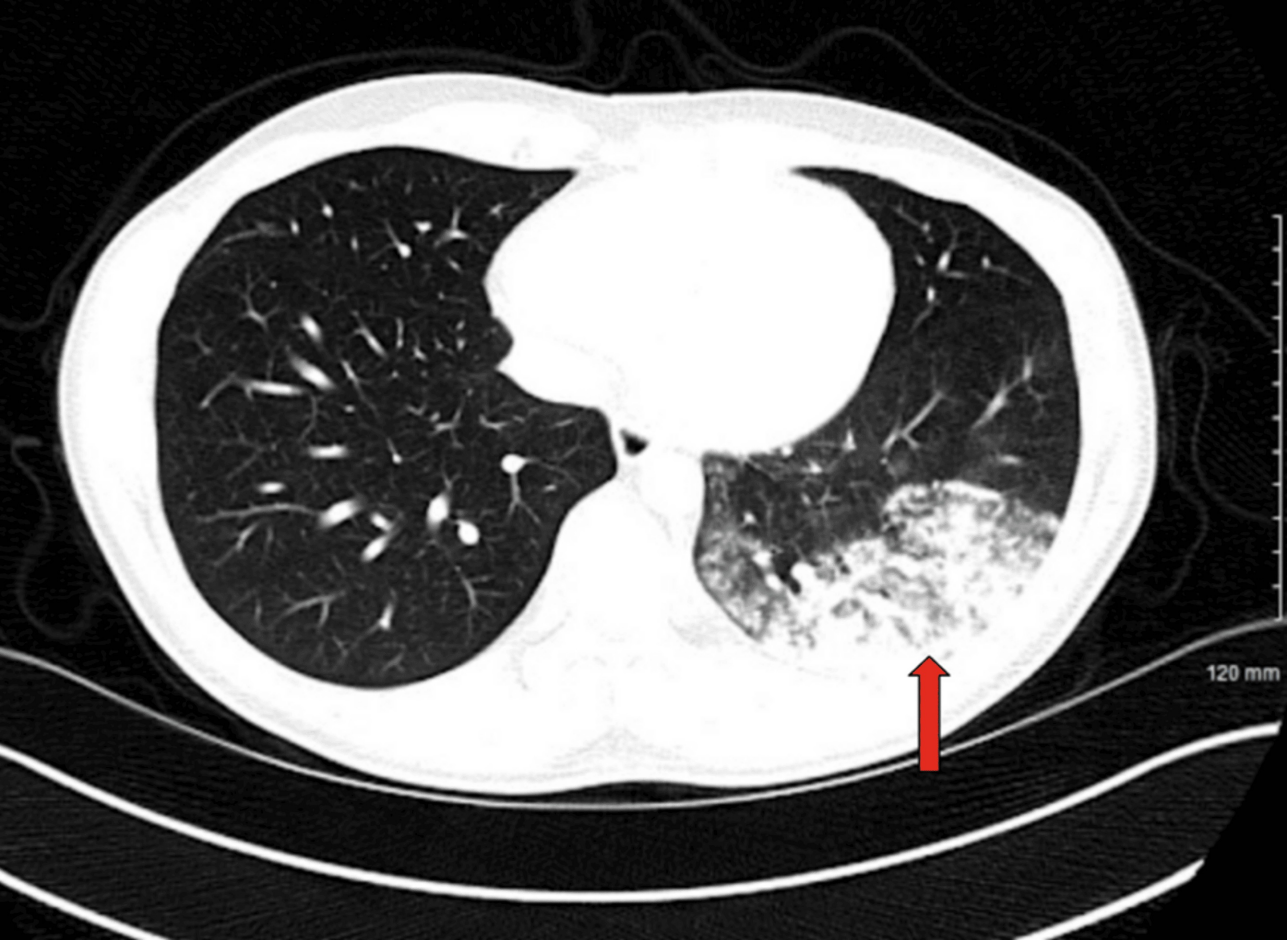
An axial computed tomography scan of the chest revealing cluster nodular and ground-glass density infiltrates with air bronchogram and necrotizing pneumonia affecting a significant portion of the left lower lobe as seen in the arrow.
